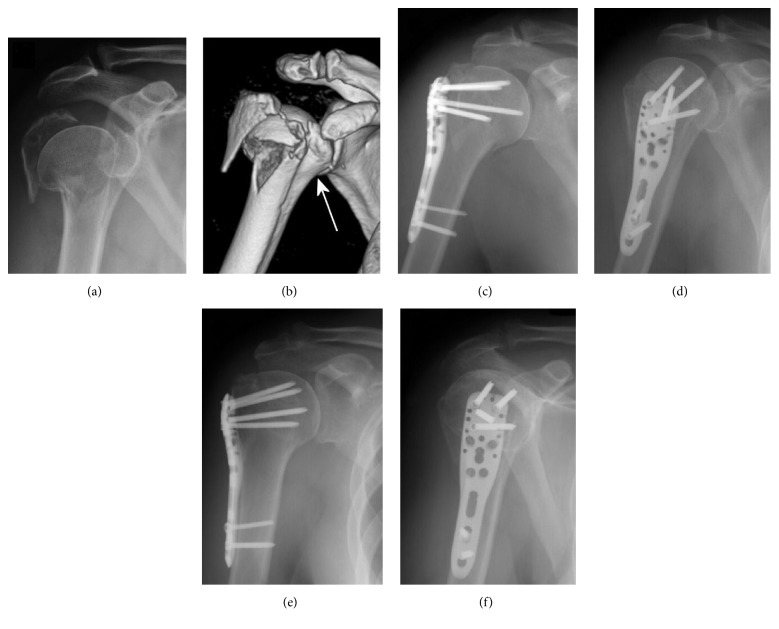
(a)-(b) Three-part valgus impacted fracture with severe displacement of the articular segment in a 48-year-old woman (AO/OTA type C2); the lesser tuberosity was in continuity with the shaft (arrow). (c)-(d) Postoperative radiograms in external (c) and internal (d) rotation showing anatomical reduction of the fracture. (e)-(f) The same radiographic views three months after surgery demonstrating union with no secondary displacement.
